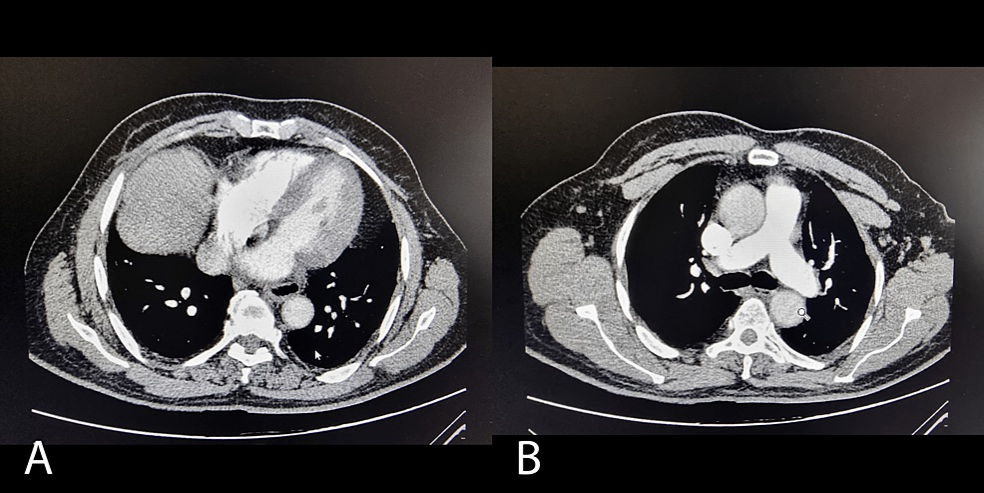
Images A and B show angio-CT scans with repermeabilization of a pulmonary embolism due to COVID-19 (after six months), respectively
